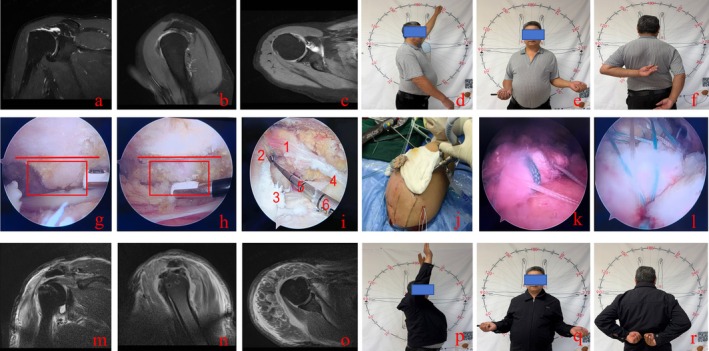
Patient, male, 68 years old, presenting with a massive irreparable rotator cuff tear. (a–c) Preoperative MRI demonstrating retraction of supraspinatus and subscapularis tears with upward displacement of the humeral head. (d–f) Preoperative shoulder joint range of motion during flexion, external rotation, and internal rotation. (g, h) Standardized acromioplasty under arthroscopic visualization during surgery. (i) 1–2: Anteroposterior diameter of supraspinatus defect medial aspect; 3–4: Anteroposterior diameter of supraspinatus defect lateral aspect; 2–5: Distance between two anchors in glenoid fossa and humeral footprint region; 2–6: Lateral diameter of supraspinatus defect area. (j–l) Intraoperative preparation of the “sandwich” patch, patch insertion into the supraspinatus defect, and rotator cuff repair. (m–o) Postoperative follow‐up MRI showing well‐repaired supraspinatus and subscapularis muscles, downward displacement of the humeral head, and good graft signal. (p–r) Postoperative follow‐up shoulder joint range of motion for flexion, external rotation, and internal rotation.
